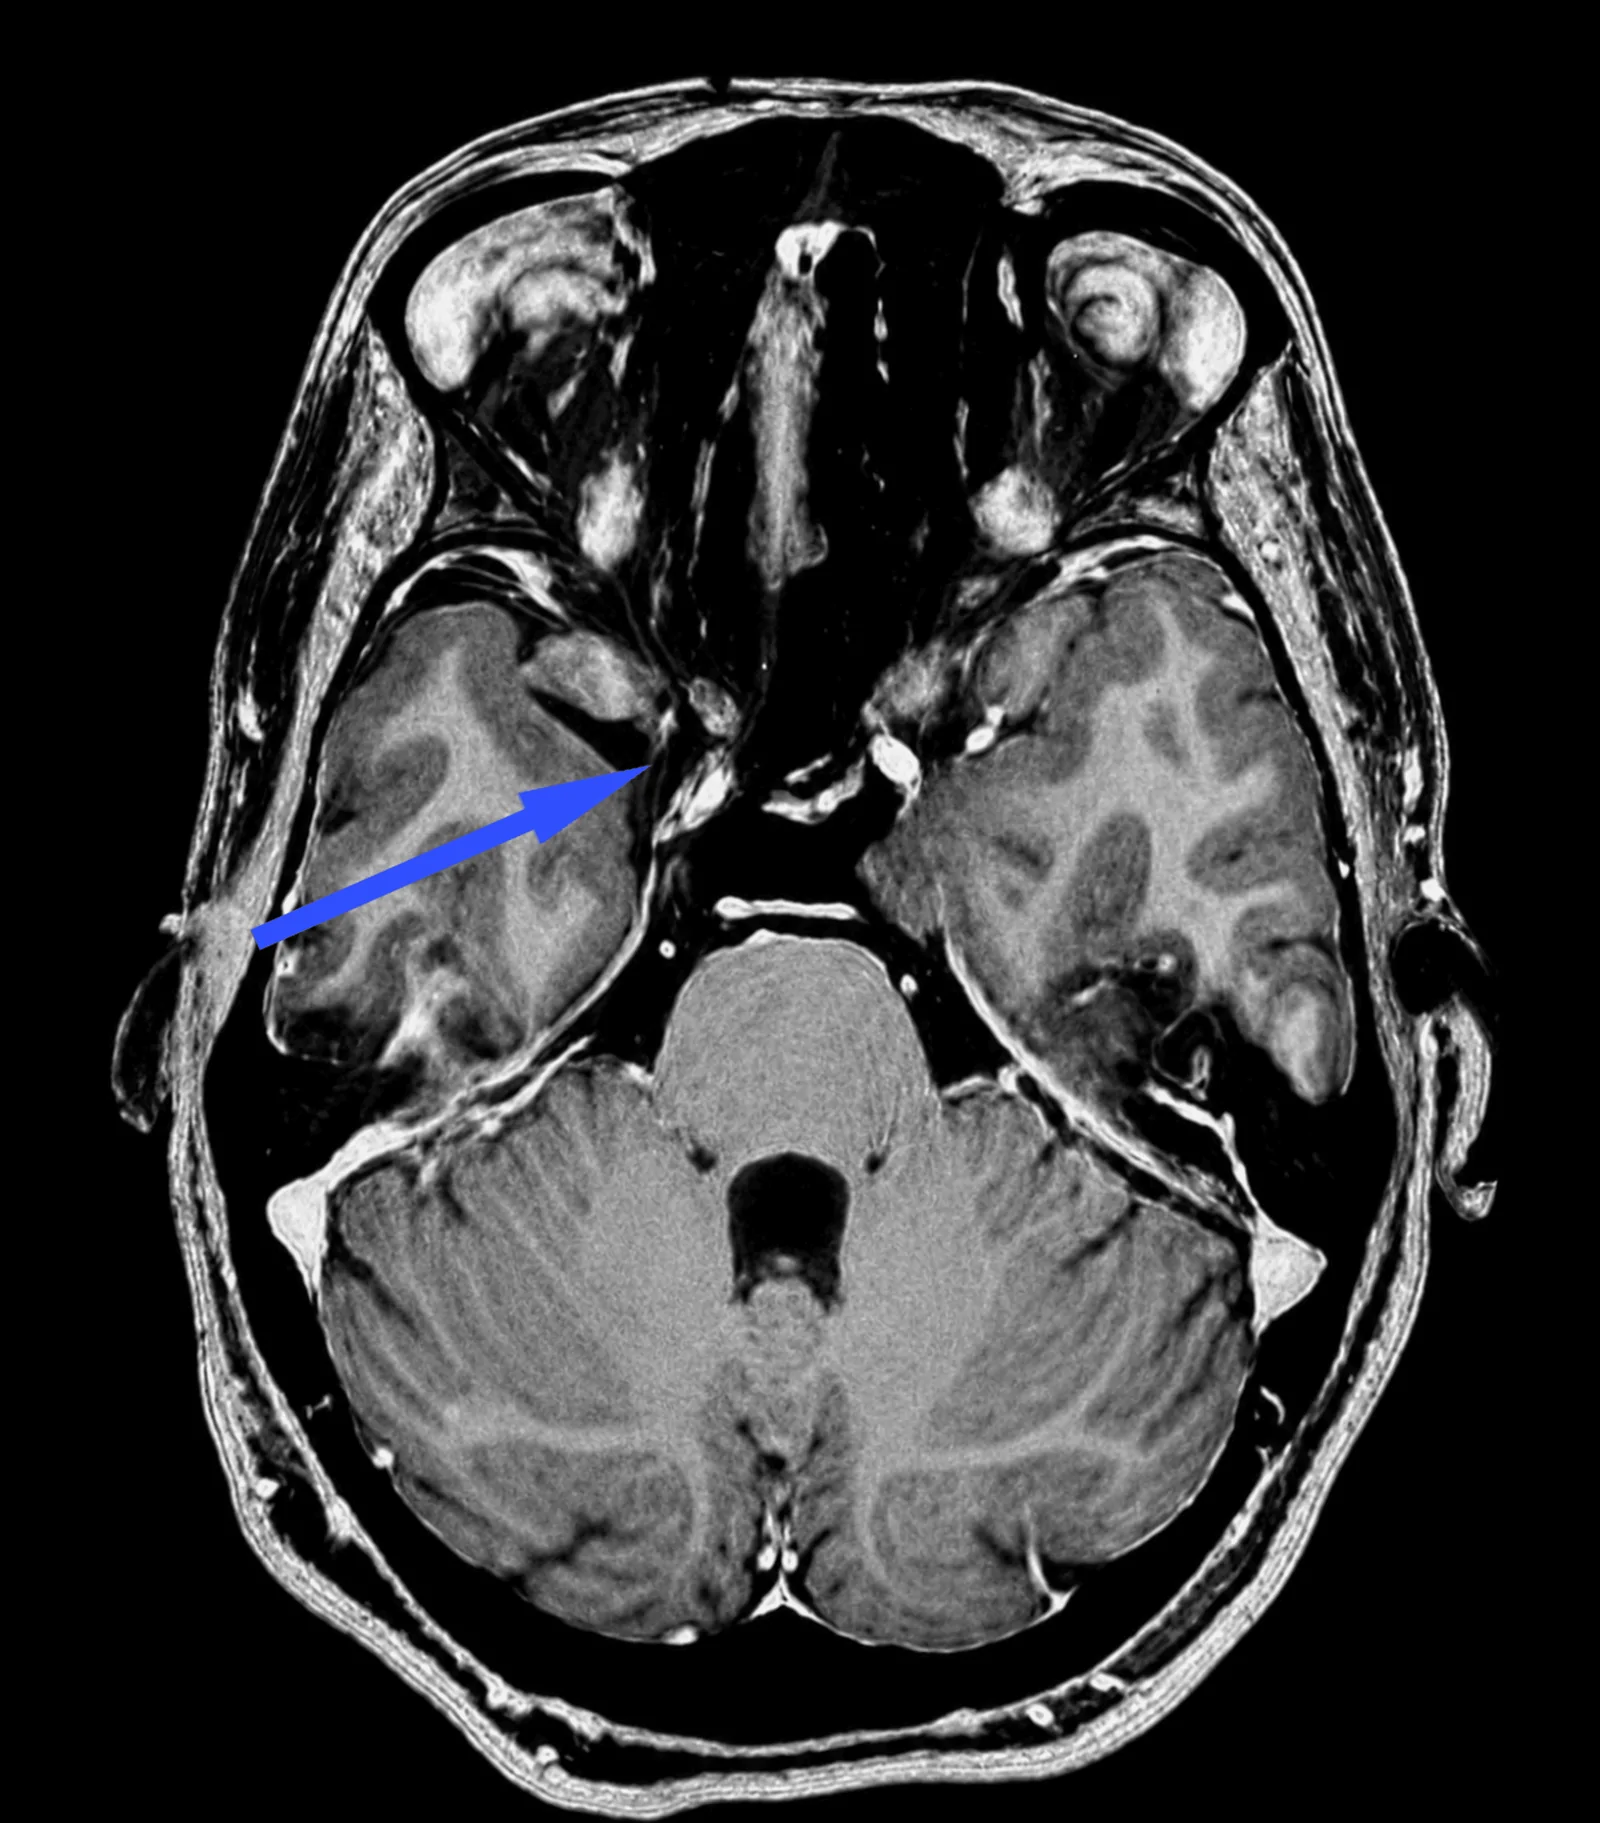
Contrast-enhanced cerebral magnetic resonance imaging of the patient. Abrupt narrowing in the P1 segment of the right posterior cerebral artery (blue arrow) with multiple collaterals along its course.
